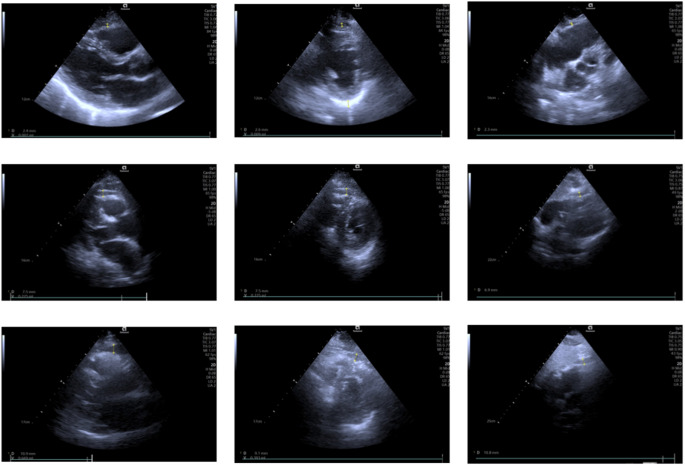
(A) Epicardial adipose tissue assessment in parasternal long axis (linear assessment), (B) Epicardial adipose tissue assessment in parasternal short axis. (C) Epicardial adipose tissue assessment
